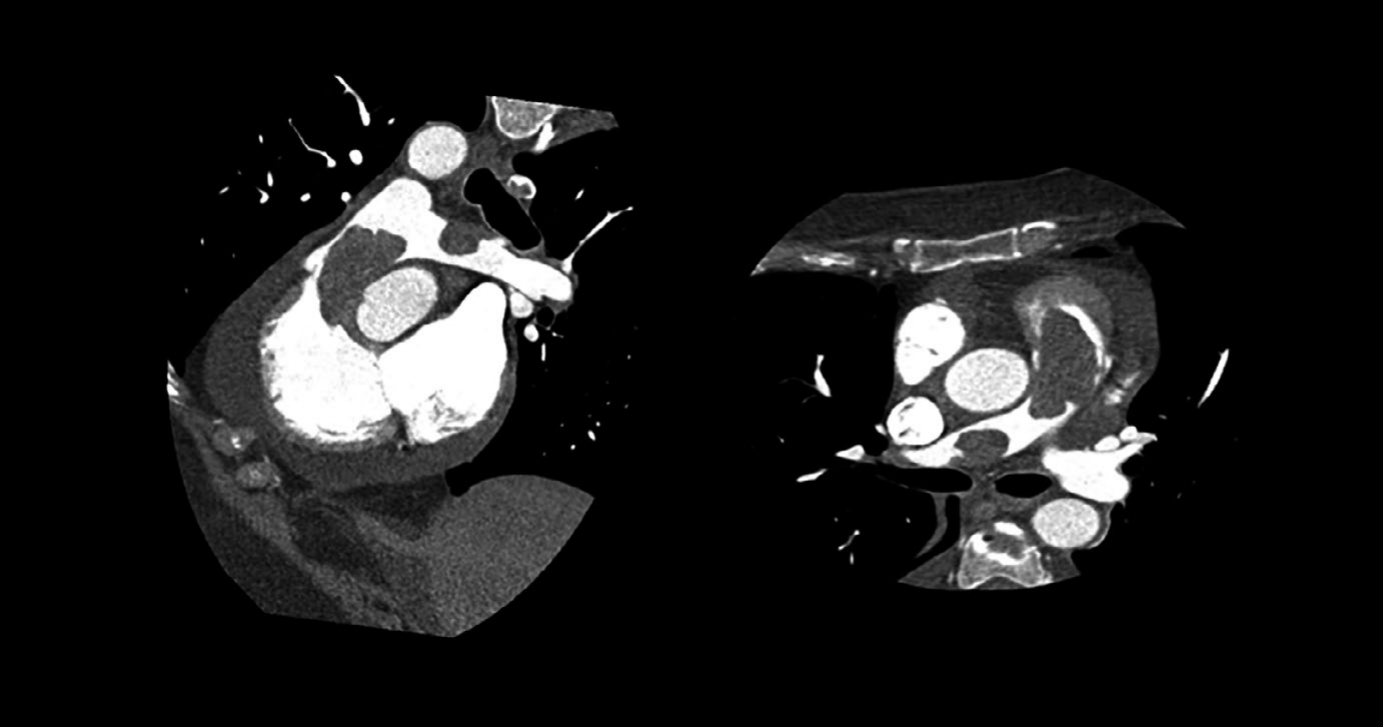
Cardiac computed tomography reconstructions showing the two masses involving the right ventricular outflow tract and the right branch of pulmonary artery
